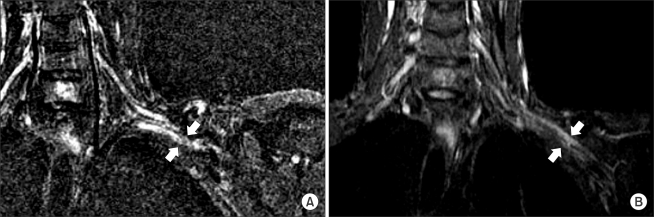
Coronal T2-weighted magnetic resonance images of the left brachial plexus. (A) The preoperative image shows that the left brachial plexus was compressed by the malunited clavicle in the costoclavicular space. (B) The postoperative image at the follow-up performed 16 months after surgery shows that the distorted brachial plexus lined up in a straight line in contrast to the alignment observed before surgery.
